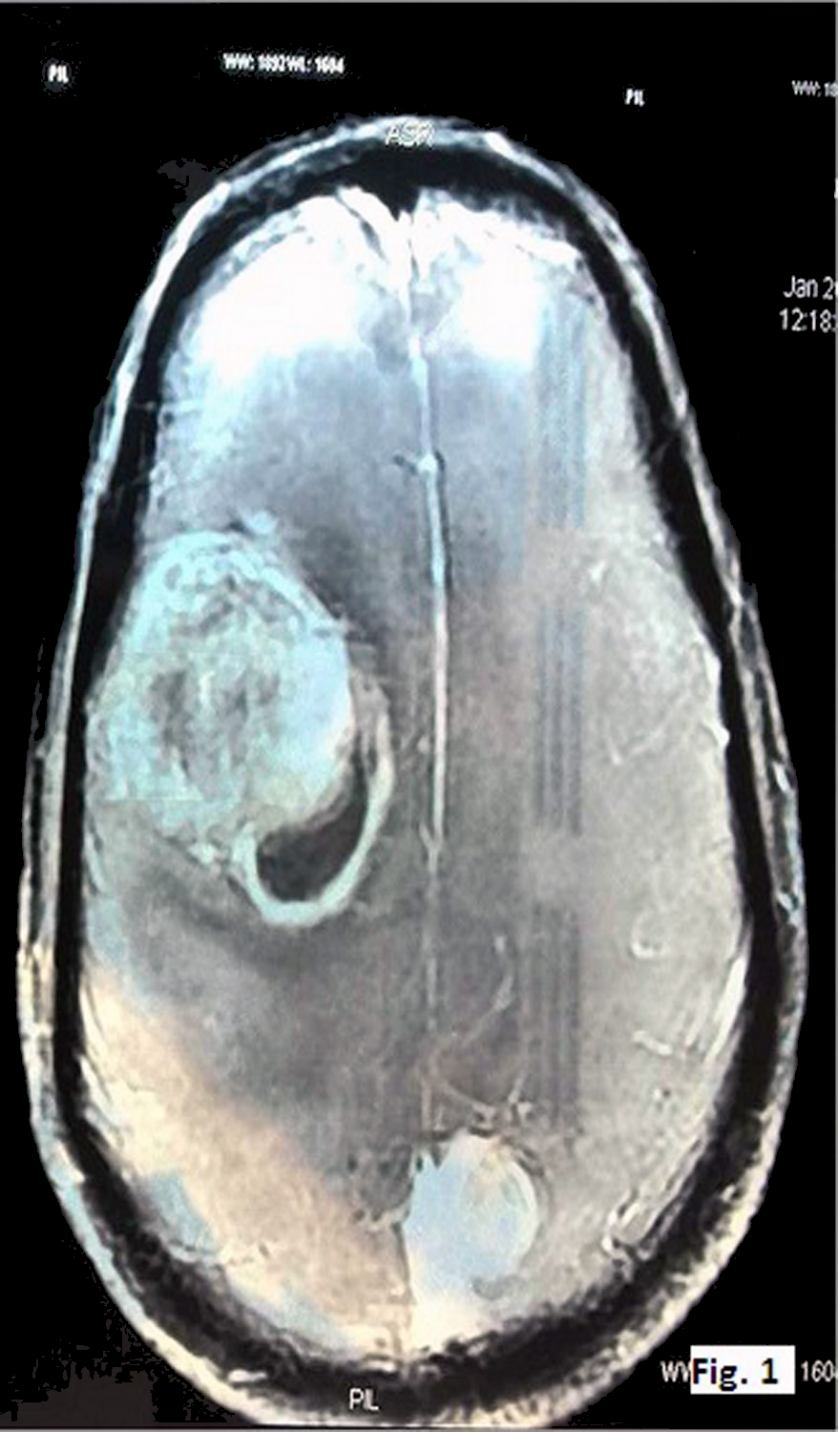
CT image showing hetero-dense lesions in both right and left parietal areas
